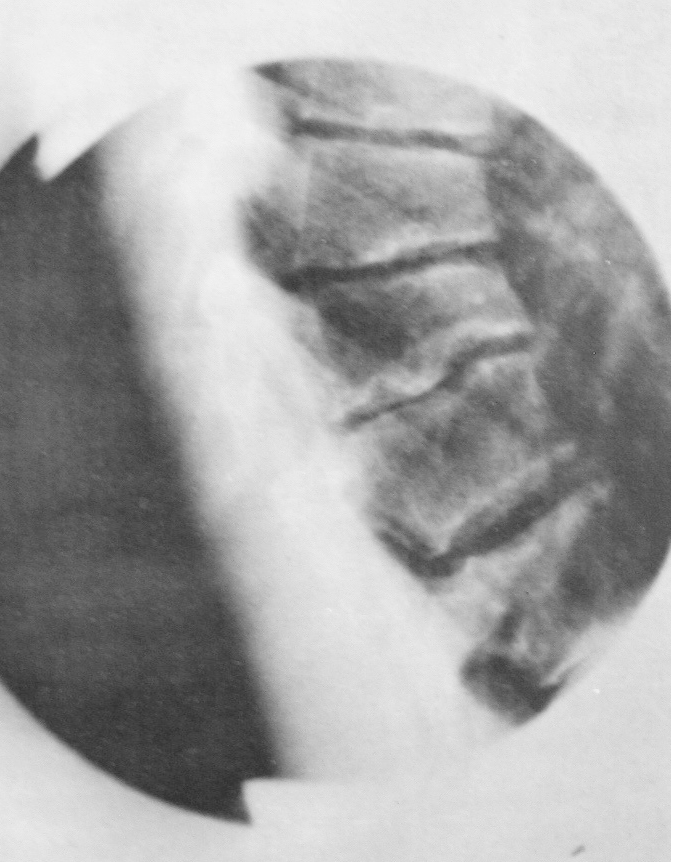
Scheuermann signs on a lateral X-ray. Characteristic radiographic findings in a patient with Scheuermann's disease in the late stage (kyphosis, wedging of vertebral bodies, endplate irregularities, Schmorl's nodes).
